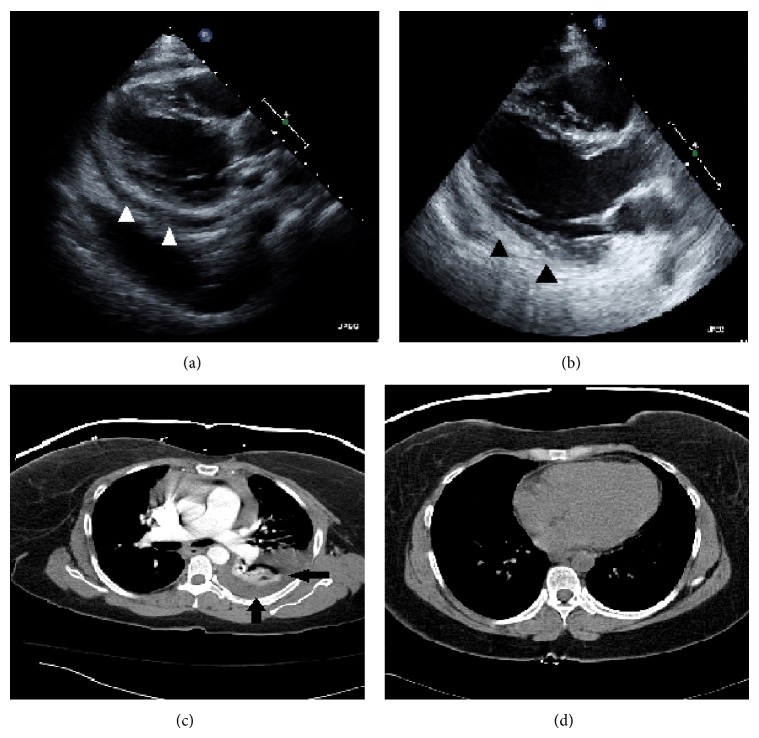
(a) Demonstrating pericardial effusion seen on echocardiogram, with (c) showing a large pleural effusion seen on computerized tomography. Both scans were obtained at second presentation in 2013. ((b) and (d)) Demonstrating complete resolution of both effusions at 3-month follow-up.
